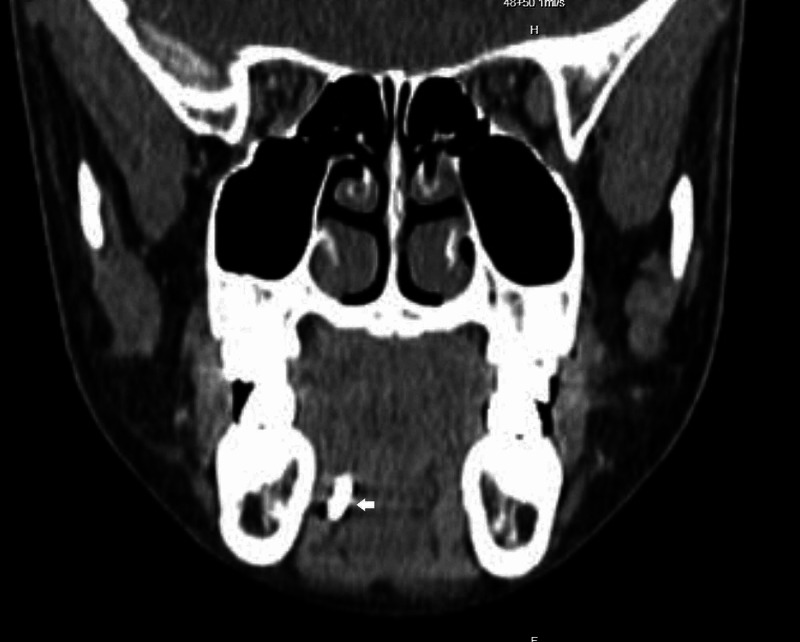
CT scan of the neck (coronal view) with a stone (white arrow) seen in the Wharton's duct
